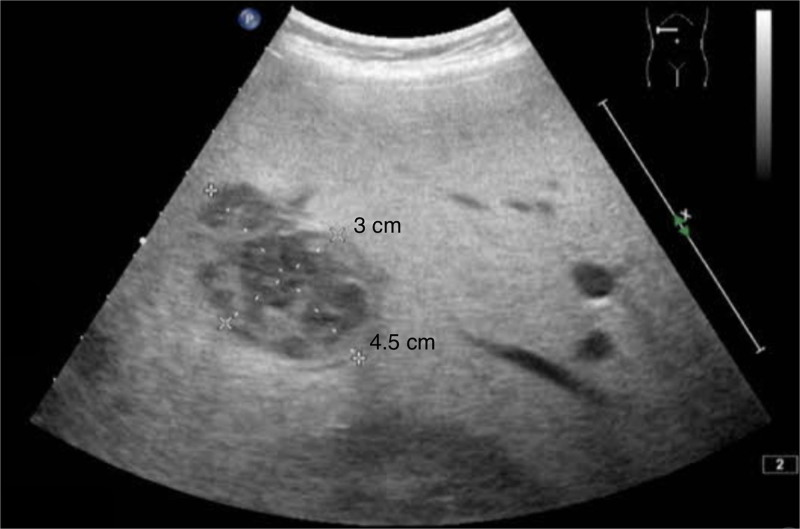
Abdominal ultrasound showing 3 × 4.5 cm multilocular cystic lesion in liver segment 6.
